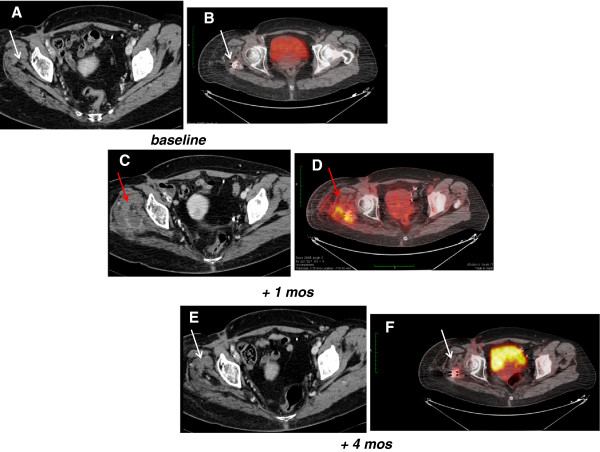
Computed Tomography scan (CT) and [18F]fluorodeoxyglucose–positron emission tomography scan (PET) abscess evaluation. CT showed a new mass in the right gluteus muscles characterized by fluid content with rim enhancement (panel C) which was not evident at baseline (panel A). That area became positive on PET (panel D) compared to baseline (panel B). CT (panel E) and PET (panel F) confirmed the resolution of the infection, three months later after sunitinib interruption and treatment with antibiotics.
